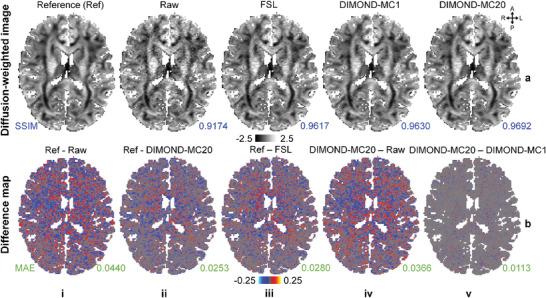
Images synthesized using tensor model. a) Exemplary axial image slices of diffusion‐weighted image (DWI) volumes (b = 1000 s mm^−2^ along [0.20, 0.89, −0.40]) from reference data (a(i), synthesized using reference tensors via the tensor model), raw acquired data (a(ii)), and those synthesized from tensors generated using FSL (a(iii)) and subject‐specific trained DIMOND (a(iv) and a(v)) from sub‐sampled data of a representative HCP subject are shown. b) Difference maps between the reference and raw acquired DWI (b(i)), reference and DIMOND‐MC20 result (b(ii)), reference and FSL result (b(iii)), DIMOND‐MC20 result and raw acquired DWI (b(iv)), and DIMOND‐MC20 and DIMOND‐MC1 results (b(v)) are shown. The structural similarity indices (SSIM, blue) are listed to quantify the similarity between displayed DWIs and the reference. The mean absolute errors (MAE, green) are listed to quantify the difference between DWIs.
